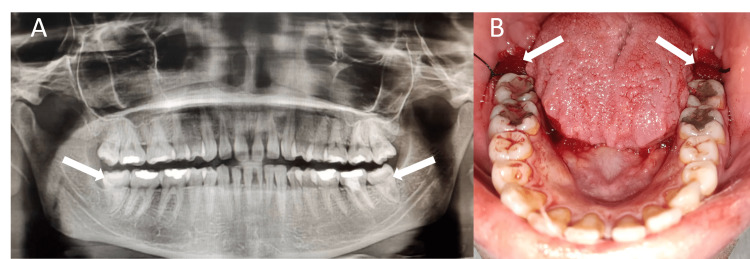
(A) Diagnostic panoramic radiograph. (B) Clinical image after the extraction of bilateral mandibular third molars and the application of ZA-loaded Gelfoam on one side and saline-loaded Gelfoam on the other side
